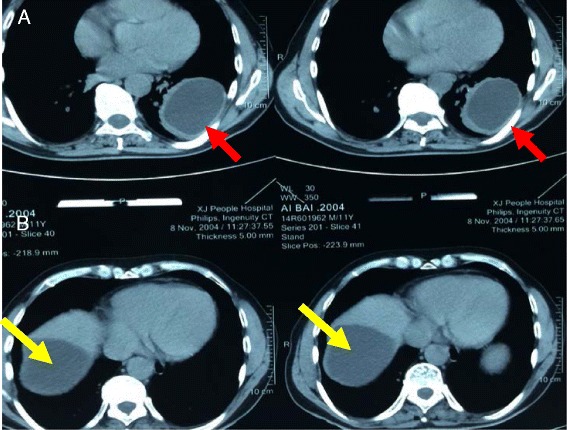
Computed tomography scan showing spherical opacity indicating the presence of a hydatid cyst in the left lower lung lobe (a, *red arrow*) and right liver (b, *yellow arrow*) of the same patient
